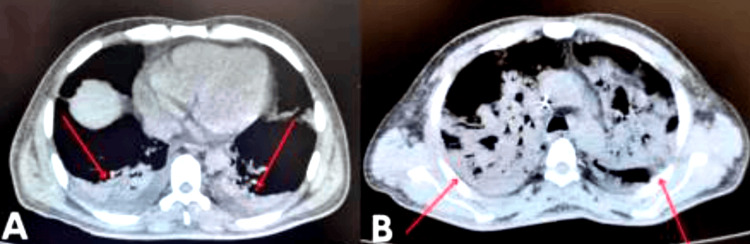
Axial slice from non-contrast computed tomography showing bilateral pulmonary complications (A) Basal consolidation secondary to an abdominal infectious process, and (B) partial pulmonary collapse associated with respiratory compromise. These findings were indicative of progression toward respiratory failure in the context of sepsis due to a liver abscess.
